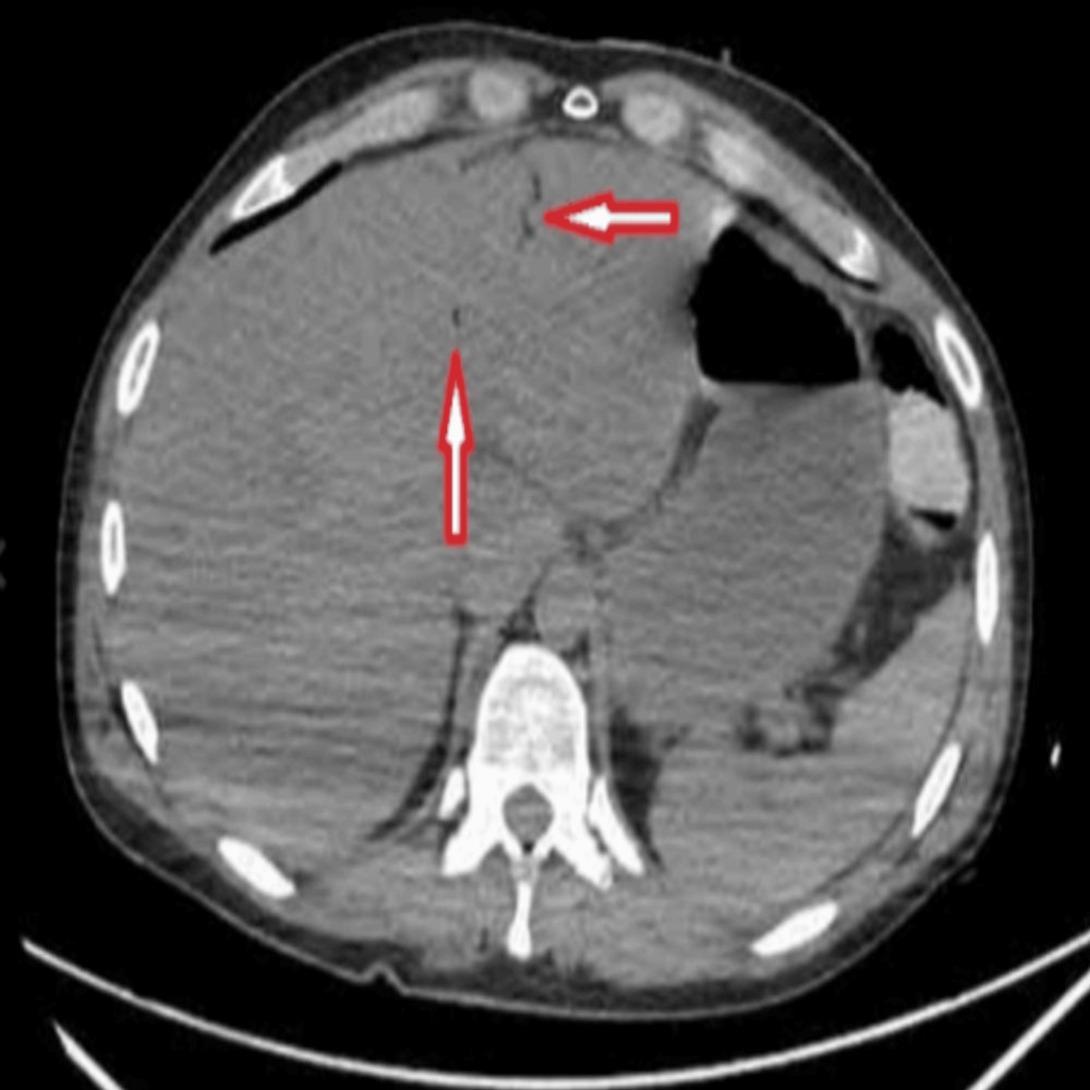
CT abdomen and pelvis, axial (transverse) section, showing portal venous gas (arrow).
